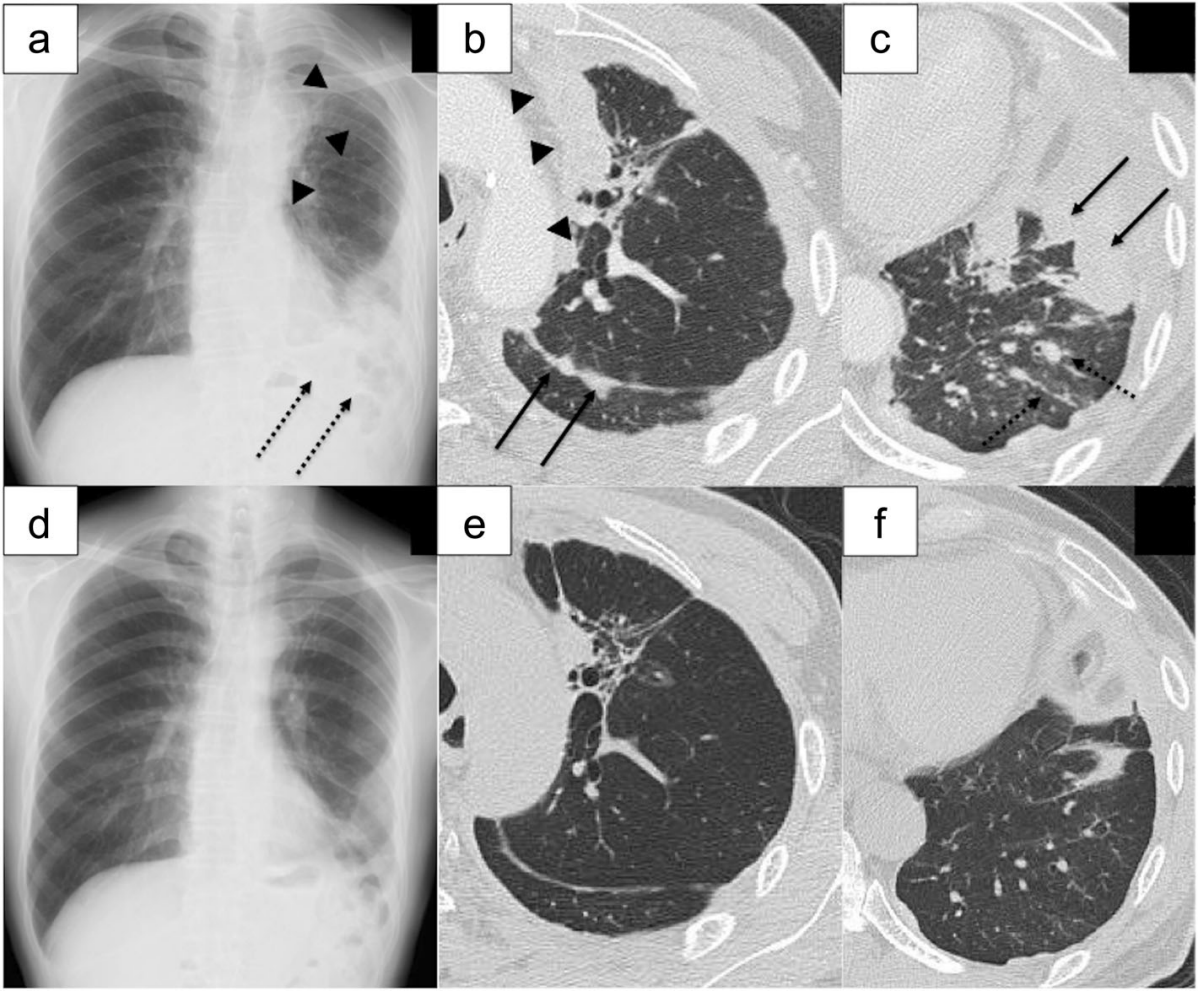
Chest imaging findings, Chest imaging at baseline (a-c), and after 13 rounds of nivolumab treatment (d-f), On the chest x-ray, primary tumor was shown in the upper lung field in contact with upper mediastinum (arrow heads), and disseminated tumor masses were mainly identified in the left lower lung field as a consolidated area (black dotted heads) (a) and were improved after nivolumab therapy (d). On the chest computed tomography image, the primary lesion in the left upper lobe adjacent to mediastinum (black arrow heads), disseminated multiple masses in the thoracic cavity (black solid arrows), and pleural and interlobular septal thickening due to lymphatic spread of tumors (black dotted arrows) (b, c) were all improved after nivolumab therapy (e, f)
